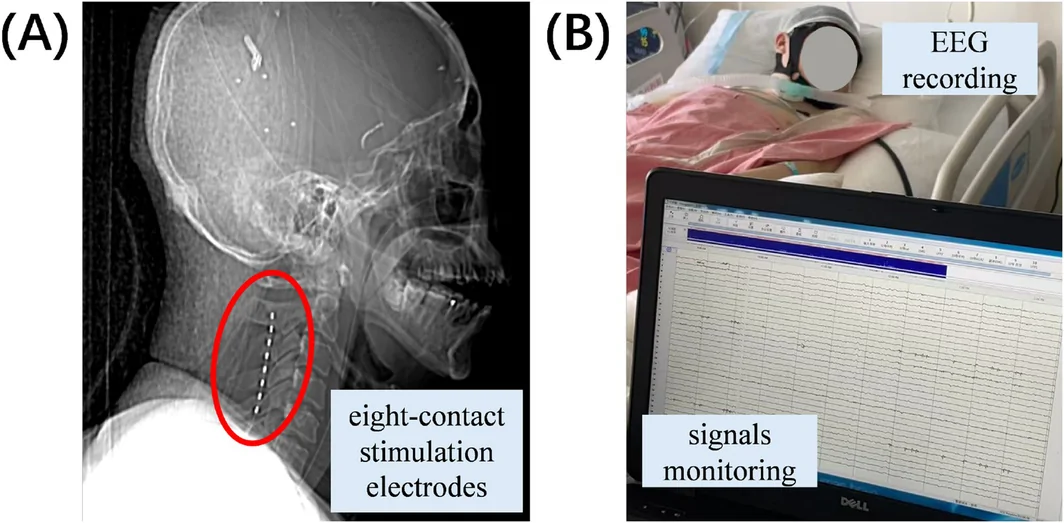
(A) Cervical CT 3D reconstruction after SCS implantation. The electrodes were advanced cranially along the midline into the epidural space and implanted at the C2 vertebrae level; (B) EEG tests were performed after surgery, including the baseline, a 15 min continuous SCS under EEG monitoring and the post‐SCS recording.
